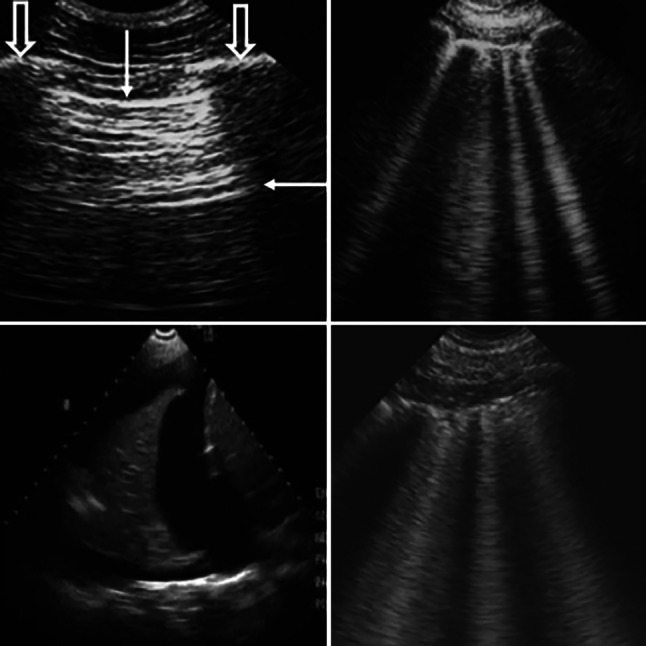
Lung ultrasound. *Upper left* Normal pattern (“dry lung”). Note the thin clear pleural line (*downwards arrow*) and two ribs with acoustic shadowing (*open downwards*
